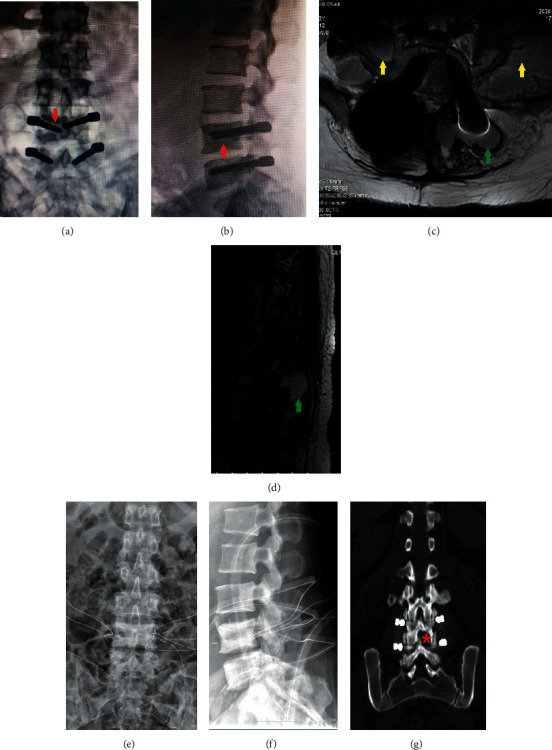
(a, b) Three years after L4-5 Dynesys dynamic internal fixation, anteroposterior and lateral X-view: the red arrow indicates bone resorption and loosening of the pedicle screw (halo sign). (c, d) Lumbar MRI: the green arrows indicate empyema around pedicle screws and spacers, and the yellow arrows indicate bilateral psoas muscle abscesses. (e, f) Placing drainage tubes after removal of the implant. (g) Opening a window between the lamina, retaining the lateral 1/2 of the inferior articular process in L4.
